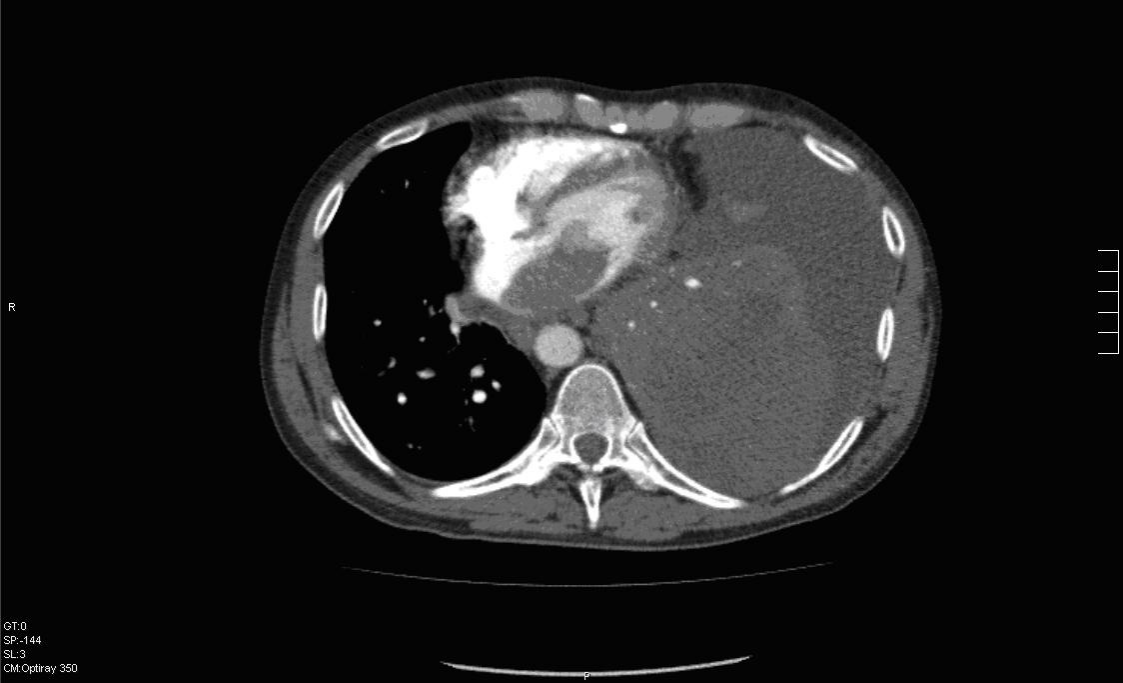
Computed tomography shows a large mass in the left pleural space.
